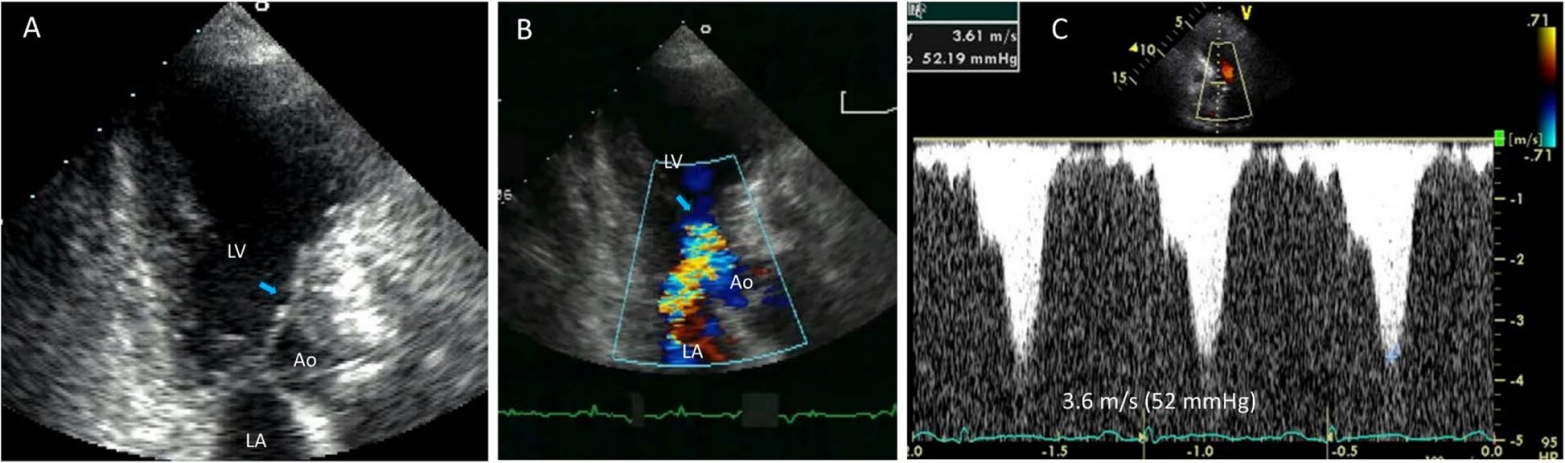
A representative case showing left ventricular outflow tract obstruction associated with Takotsubo syndrome. A 68-year-old woman developed severe dyspnea after uneventful bronchoscopy. A Echocardiography showed typical apical ballooning and prominent systolic contact of the mitral valve and ventricular septum (arrow) resulting in (B, C) marked flow acceleration and left ventricular outflow tract obstruction
